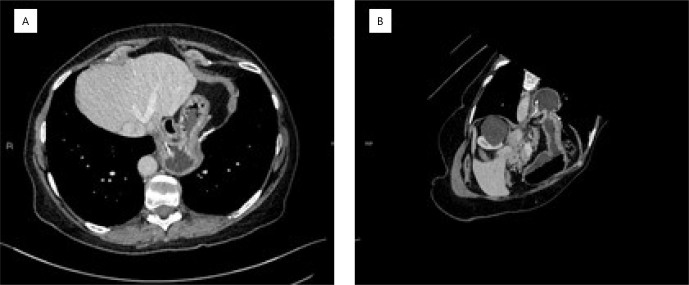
Computed tomography of the abdomen: partial mesh erosion into the gastric wall. (A) Axial section and (B) sagittal section.
